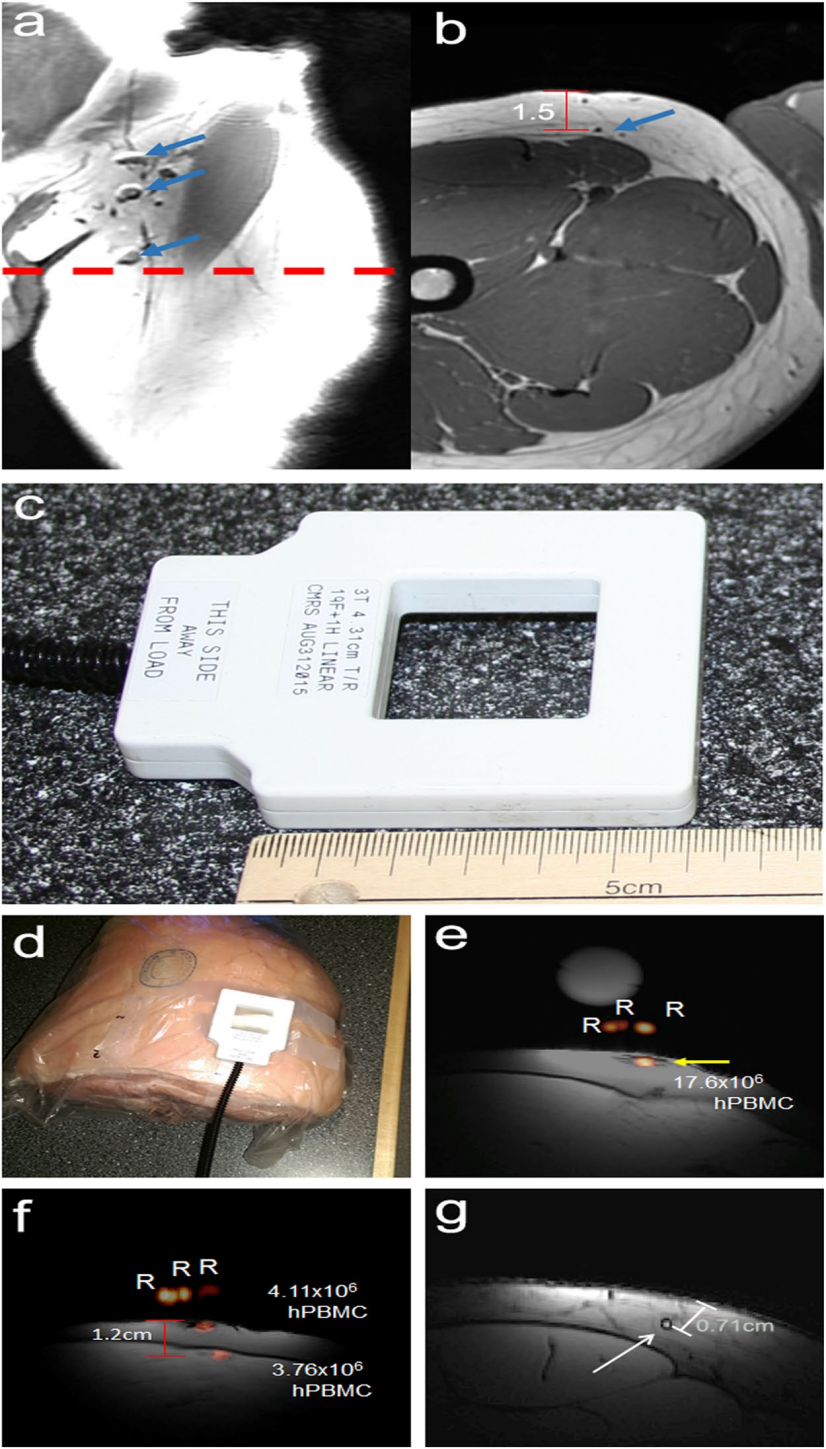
A clinical 3 T MRI scanner detects ^19^F-PFC-labeled human PBMC. (a) A T1-weighted, coronal orientation MR image obtained using a body coil reveals human inguinal lymph nodes as dark spheres (blue arrows) within the fat of the upper thigh. The red dashed line indicates the location of the axial MRI slice (b). In the axial image, the position of the lymph node is measured to be approximately 1.5 cm below the skin (b, red scale bar). (c,d,e,f,g) PBMC were obtained from a healthy volunteer and labeled overnight with the ^19^F-PFC cell-labeling agent before injection into a ham shank. Imaging was performed by placing the ^1^H/^19^F switchable RF (c) coil on the ham shank as shown (d). Cells were administered intradermally with MR images shown for the, 20 × 10^6^ (e, yellow arrow) and the 4.5 × 10^6^ (f, yellow arrow) intradermal doses. An additional 4.5 × 10^6^ dose was administered intramuscularly (f, yellow arrow), which is visible at a 1.2 cm depth as measured from the MR image. A representative human proton image taken with the same parameters closely resembles the ham images in both tissue appearance and subcutaneous fat deposition (g). Scale bars represent 6 cm.
